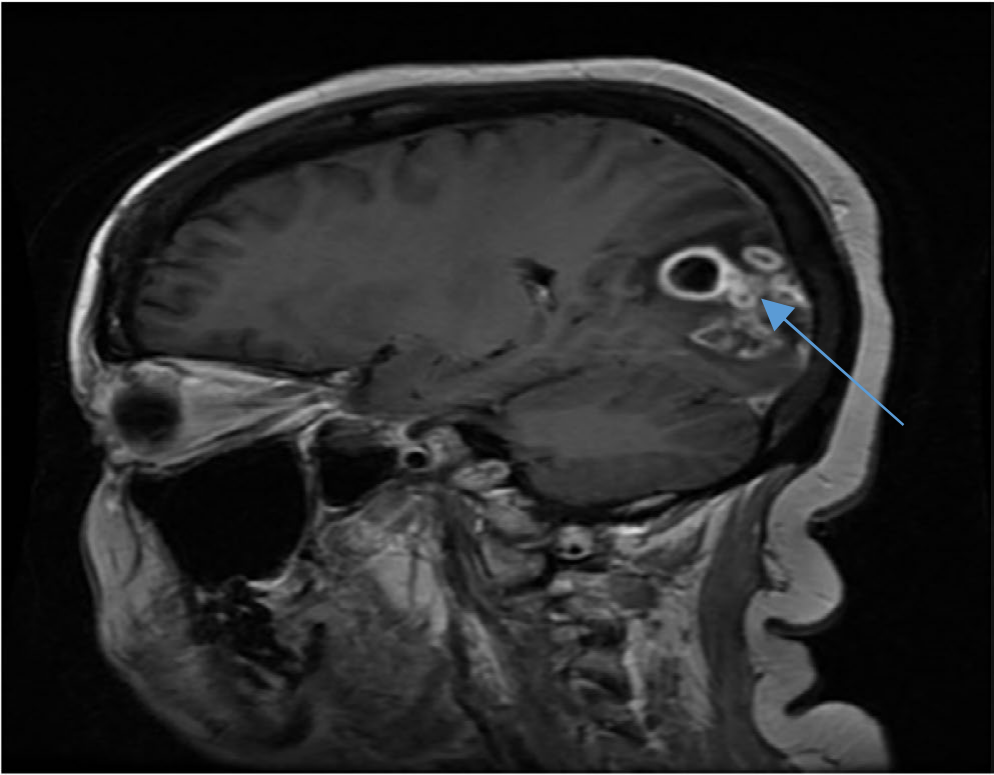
Cranial sagittal T1‐weighted magnetic resonance image showing an abscess formation in the right occipital region (blue arrow)
